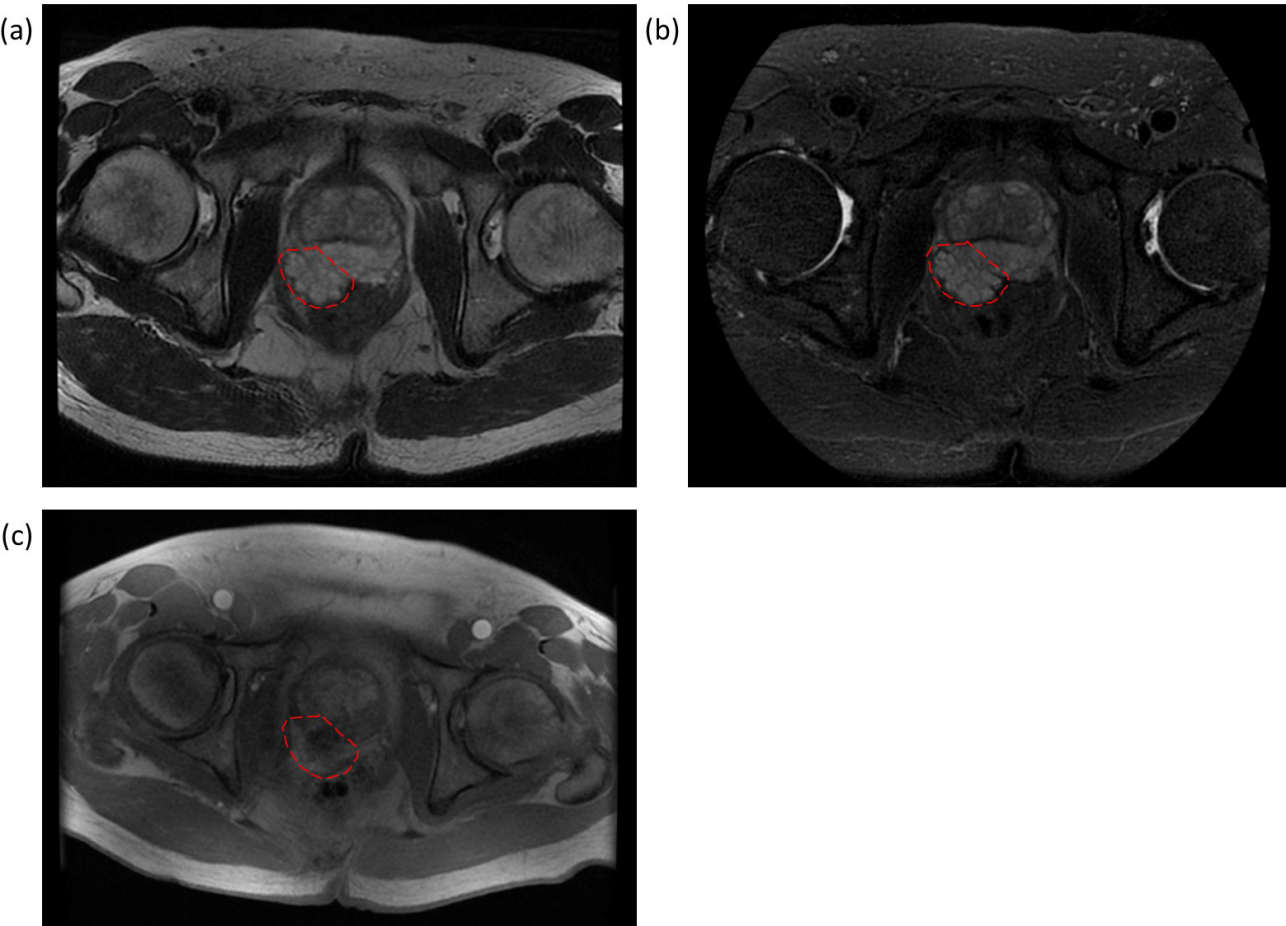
Pelvic MRI of enlarged prostate with dorsal irregular mass. The prostate is enlarged with a local protrusion towards the bladder (6 cm×5 cm×6 cm). An irregular mass is observed on the dorsal side of the prostate, closely associated with the peripheral zone, with lobulated margins and poorly defined borders adjacent to the anterior rectal wall. Red dashed circles indicate the approximate location of the lesion. (a) Axial T2-weighted image: the lesion appears hyperintense. (b) Axial fat-suppressed T2-weighted image: the lesion remains hyperintense. (c) Axial contrast-enhanced T1-weighted image: the lesion demonstrates heterogeneous enhancement.
